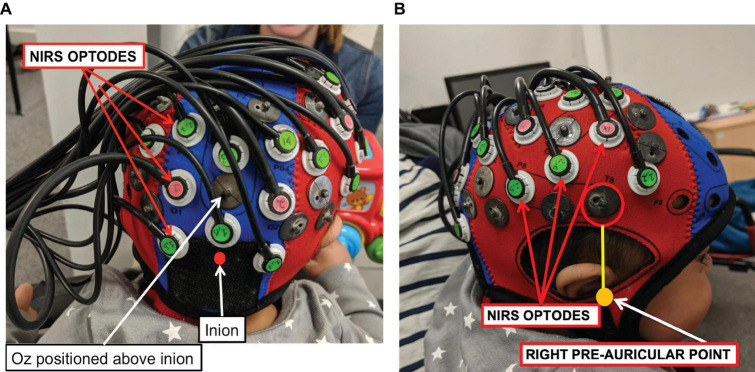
A NIRS-MRI age-appropriate co-registration map (Lloyd-Fox et al., 2014) was used to align the lower front of the array (marked with a red circle) with the right pre-auricular anatomical landmark. The bNIRS optodes are indicated, the green optodes are detectors while the pink ones are sources. Panel (A) shows the posterior view and panel (B) shows the lateral view.
